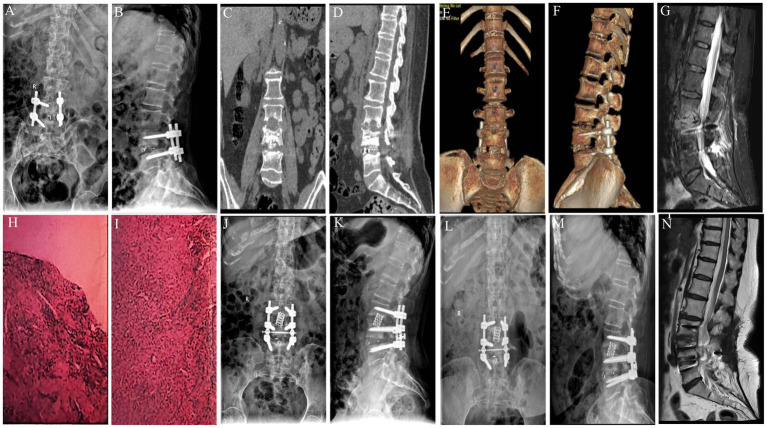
(A,B) Anteroposterior and lateral radiographs of the L4–L5 vertebral body fusion surgery. (C–F) A lumbar CT plain scan with three-dimensional reconstruction following L4–L5 vertebral body fusion surgery, which suggests bone destruction of the L3-L5 vertebrae, with soft tissue swelling consistent with infection. (G) The enhanced MRI T2WI sequence of the lumbar spine after L4-L5 vertebral fusion surgery, which indicates the postoperative changes of the L4 and L5 vertebral bodies and the abnormal signals of the L3-L5 vertebral bodies and intervertebral discs, considering the postoperative infection of the lumbar spine. (H,I) The pathological findings of L3-L5 vertebral lesion tissue, which showed inflammatory granulation tissue, necrotic tissue, fibrous tissue, and fibrocartilage tissue, with numerous chronic inflammatory cell infiltrates. (J,K) The anteroposterior and lateral X-ray films at 1 month after lumbar lesion clearance and lumbar interbody refusion. (L,M) The anteroposterior and lateral lumbar X-ray films at 4 months after lumbar lesion clearance and lumbar interbody refusion. (N) The lumbar MRI T2WI sequence at 6 months after lumbar lesion clearance and lumbar interbody refusion, which indicates that the signal intensity of L3-L5 intervertebral discs was reduced to varying degrees, the internal fixation device was in place, the interbody fusion was good, and the vertebral body was not damaged.
